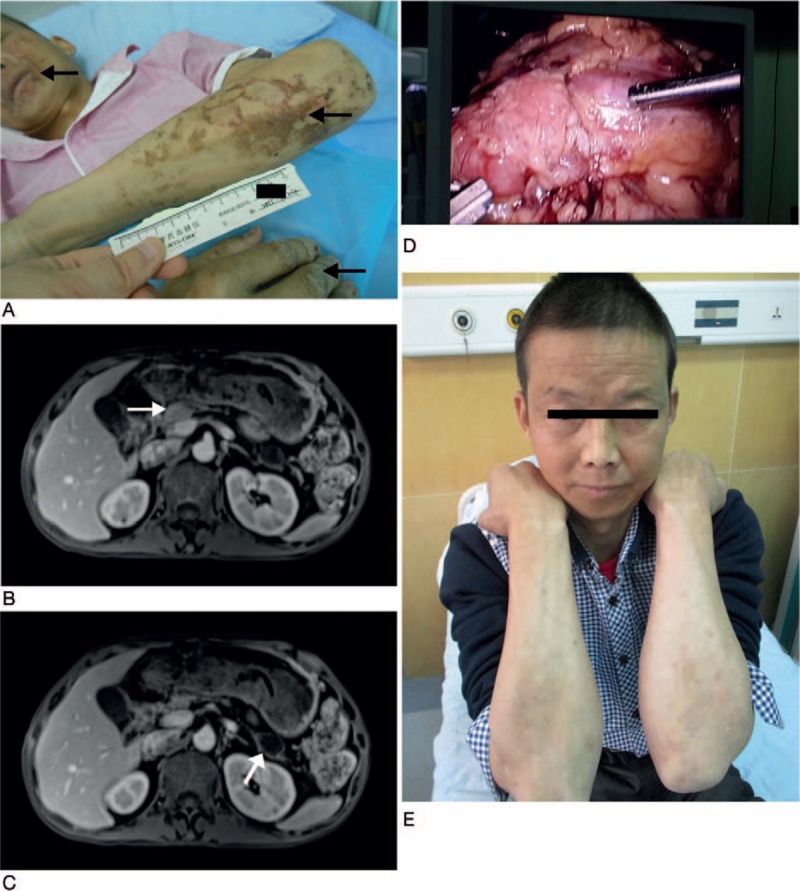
(A) Ulcerated skin rash on the upper extremities and perioral skin (black arrow). (B, C) Abdominal enhanced MRI reveals a suspected mass lesion in the head (white arrow) and a cystic lesion in the tail of the pancreas (white arrow), respectively. (D) The intraoperative tumor image is shown. (E) Within 4 wk after surgery, the skin manifestations spontaneously resolved.
